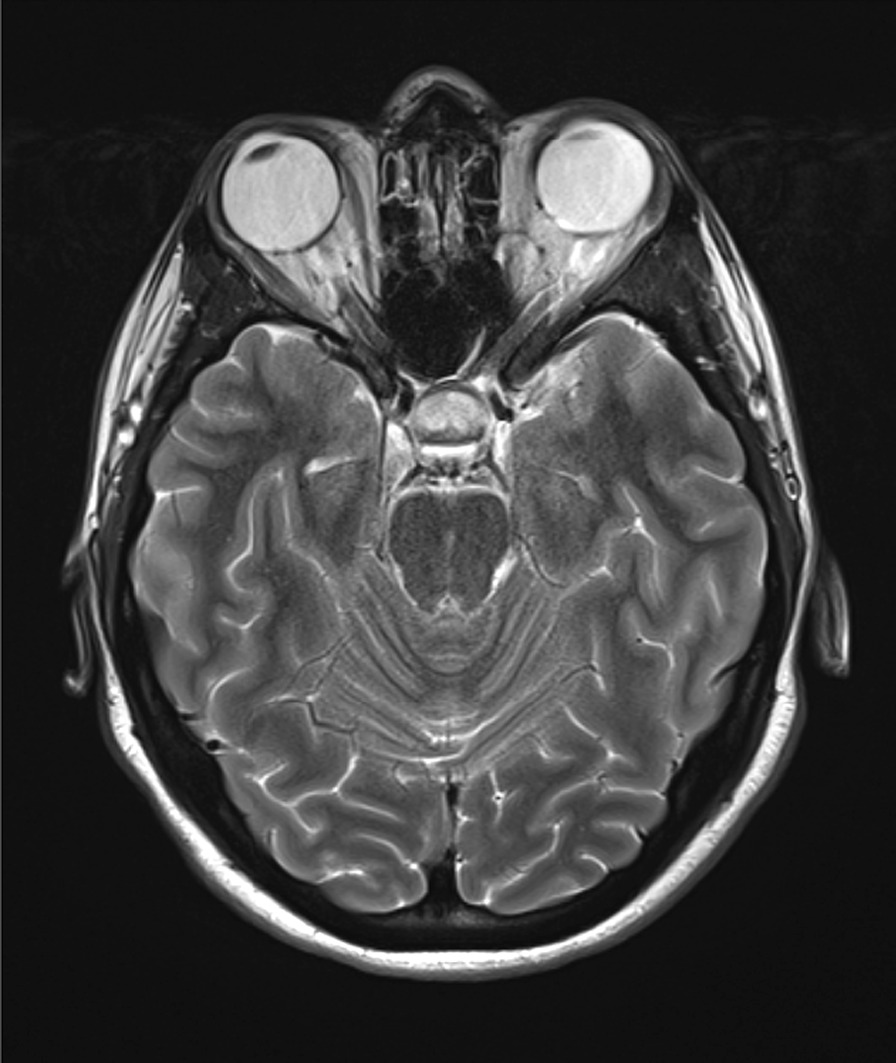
Pre-operative MRI brain scan axial T2 sequence showing a hyperintense pituitary mass
